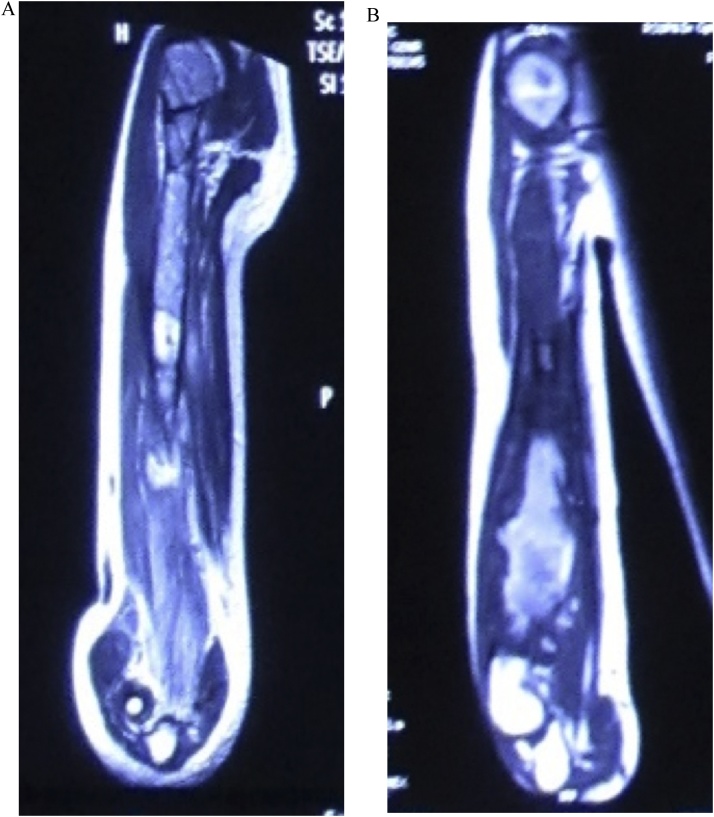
MRI of the humerus. A. Initial state, B. Post neoadjuvant chemotherapy.
